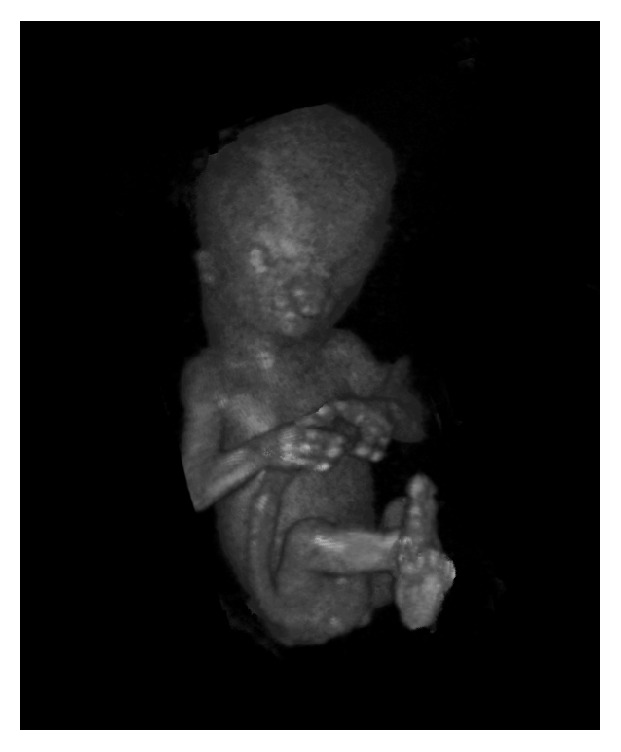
3D transvaginal ultrasound dataset of a fetus with an ectrodactyly ectodermal dysplasia-cleft (EEC) syndrome visualized in Virtual Reality. Bilateral split hands and split feet are seen as well as bilateral cheilognatoschisis. An overriding aorta with a ventricle septum defect was diagnosed additionally.
